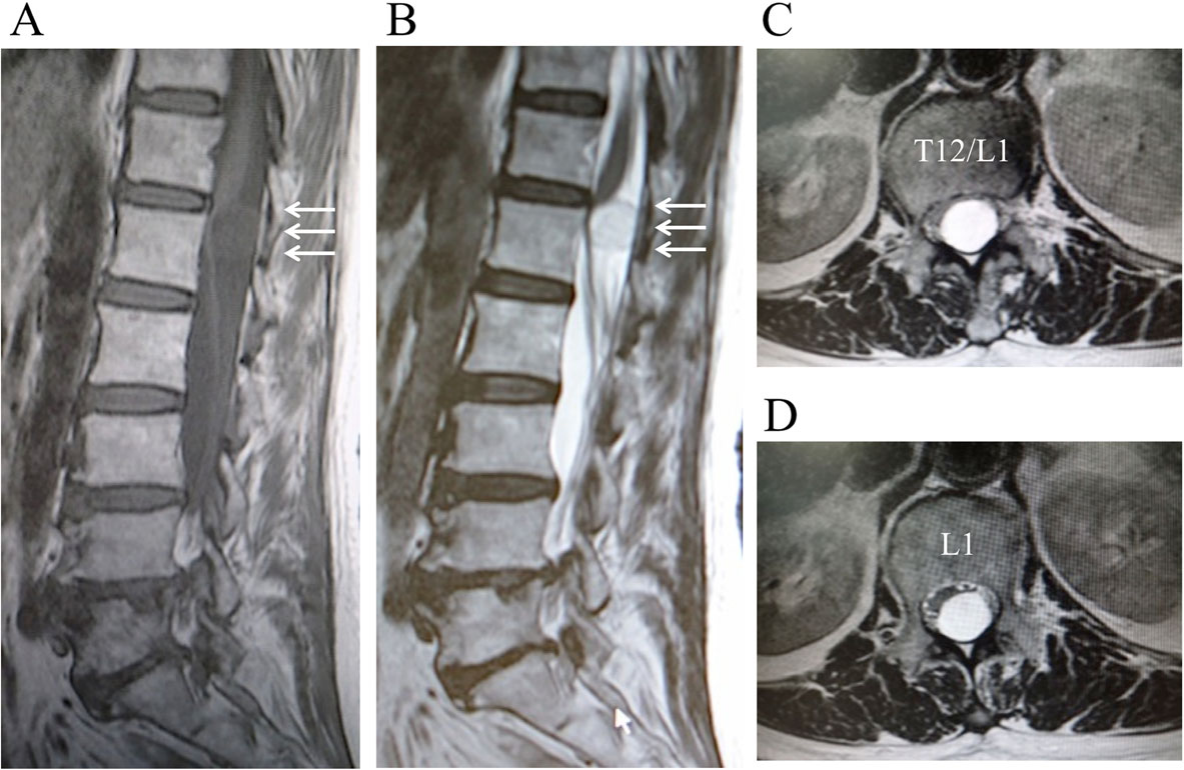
Magnetic resonance imaging (MRI) of the thoracolumbar spine preoperatively. MRI of the thoracolumbar spine showing an intradural extramedullary spinal cord tumor at the T12–L1 (*white arrows*). Views: a T1-weighted sagittal, b T2-weighted sagittal, c T2-weighted axial at T12–L1, and d T2-weighted axial at L1. The tumor was hypo-intense on a T1-weighted view and hyper-intense on a T2-weighted view. The spinal cord was markedly shifted anteriorly by the tumor
